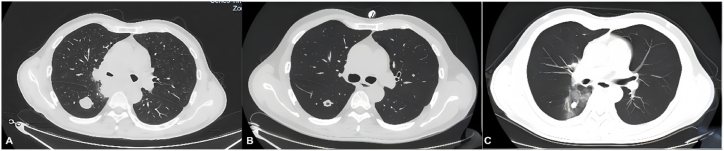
Serial contrast-enhanced CT scans. (A) May 2024: 2.14 × 2.45 cm lobulated mass in right lower lung with mediastinal/hilar lymphadenopathy. (B) September 2024: Tumor reduced to 1.6 × 1.2 cm with cavitation after immunochemotherapy. (C) February 2025: Further reduction to 1.4 × 1.0 cm, cavitation persisted, no new metastases.
